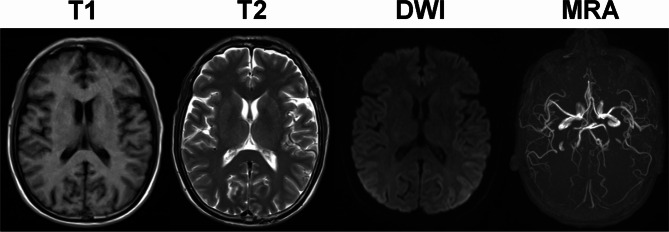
Brain MRI (T1, T2, DWI, MRA) scan on Day 2 did not show new infarct
